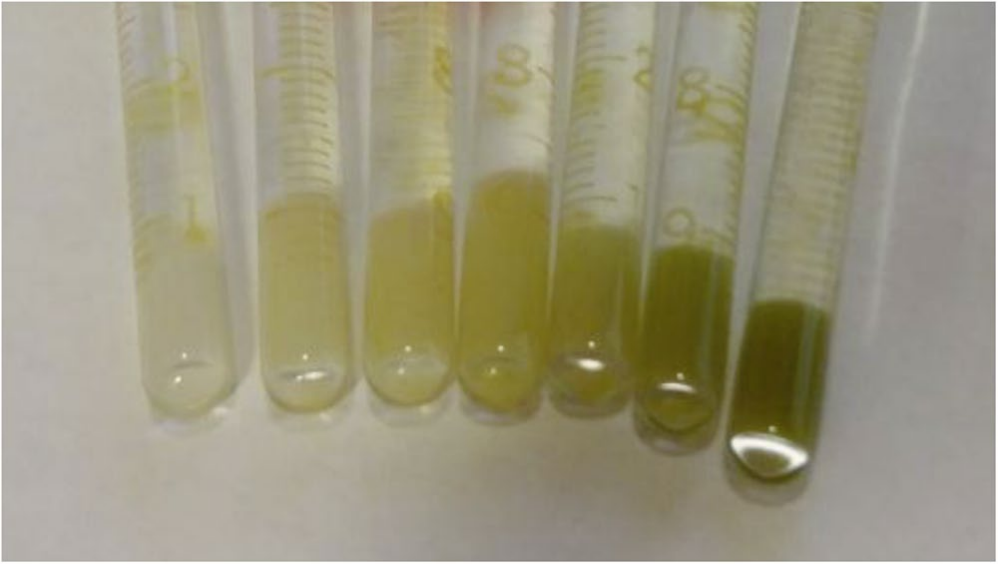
Labeled human embryonic stem cells prepared for MR imaging. Cell pellets in borosilicate glass tubes show characteristic dark green of the porphyrin contrast agent. A darker green corresponds to increased contrast uptake.
